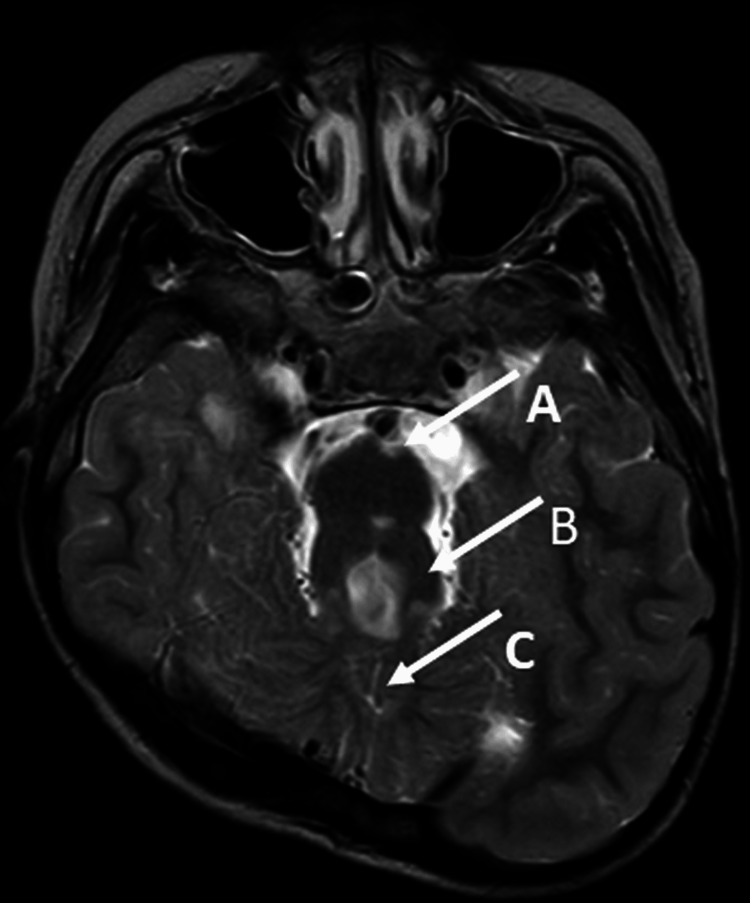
T2-weighted axial images on MRI of child 2 The MRI image was taken when he was nine years of age. The "Molar tooth sign" image is characterised by a deep posterior interpeduncular fossa (white arrow A), thickened and elongated superior cerebellar peduncles (white arrow B), and hypoplasia or agenesis of the cerebellar vermis (white arrow C). MRI: magnetic resonance imaging
